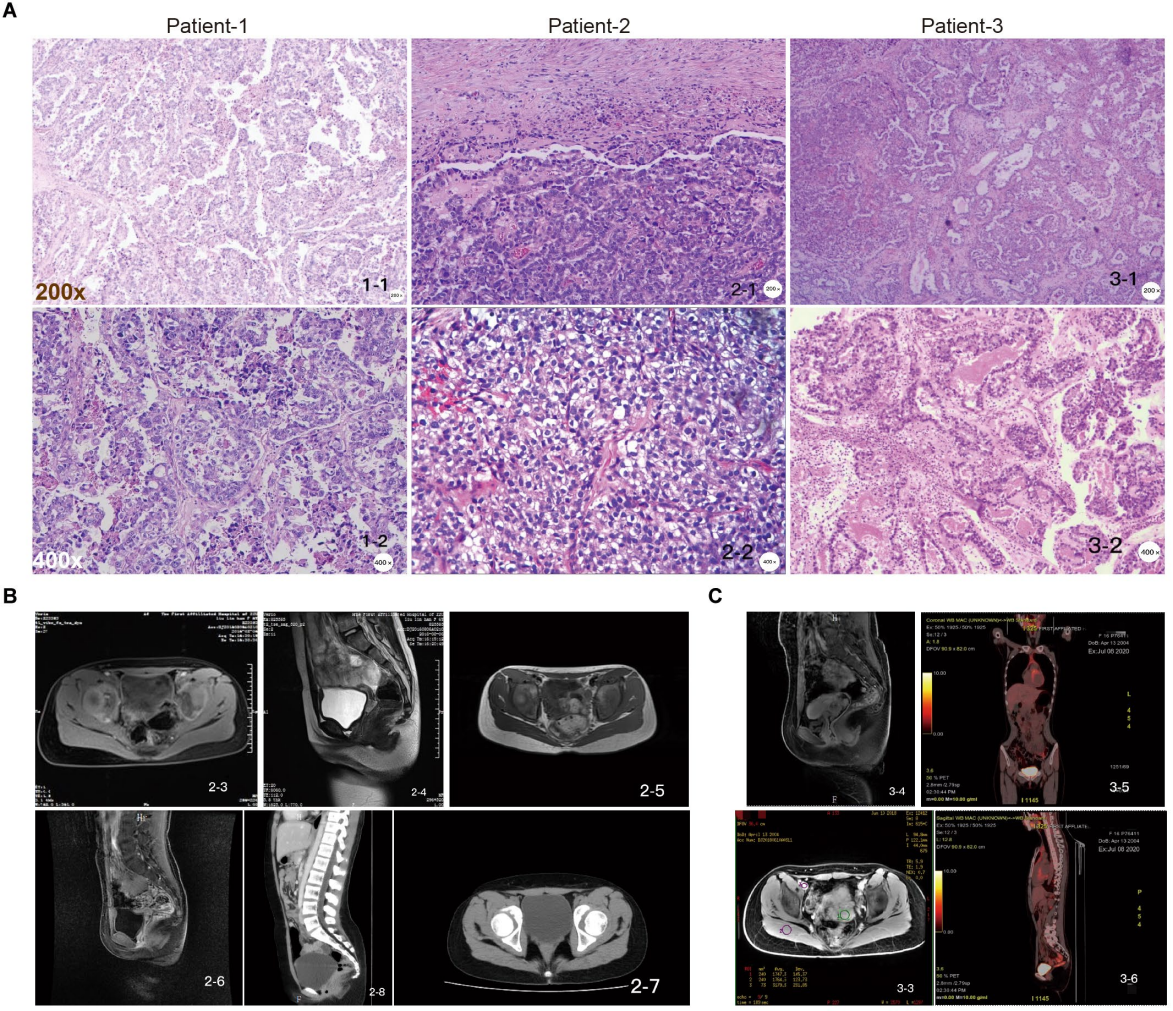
Pathologic examination of juvenile CCAC independent of HPV. (A) Patient-1, papillary growth areas with relatively small and regular papillae, sometimes larger papillae, with fibrovascular axis, tumor composed of moderately to severely atypical cells with clear cytoplasm and polygonal cells; patient-2, the tumor was composed of moderately to severely atypical cells with clear cytoplasm and polygonal cells; patient-3, in the papillary growth area, the papilla are small and relatively regular, sometimes larger, with fibrovascular axis, “shoe spike” cells, and cells protruding into the lumen of the gland. Scale: top row: 200×, bottom row: 400×. (B) Pelvic MRI images of patient-2 at initial diagnostic stages (2-3, 2-4); suggestive of recurrence stages (2-5, 2-6); and follow-up CT images showing no signs of recurrence (2-7, 2-8). (C) Pelvic MRI images of patient-3 at initial diagnosis (3-3, 3-4); follow-up PET-CT images showed no signs of recurrence (3-5, 3-6).
